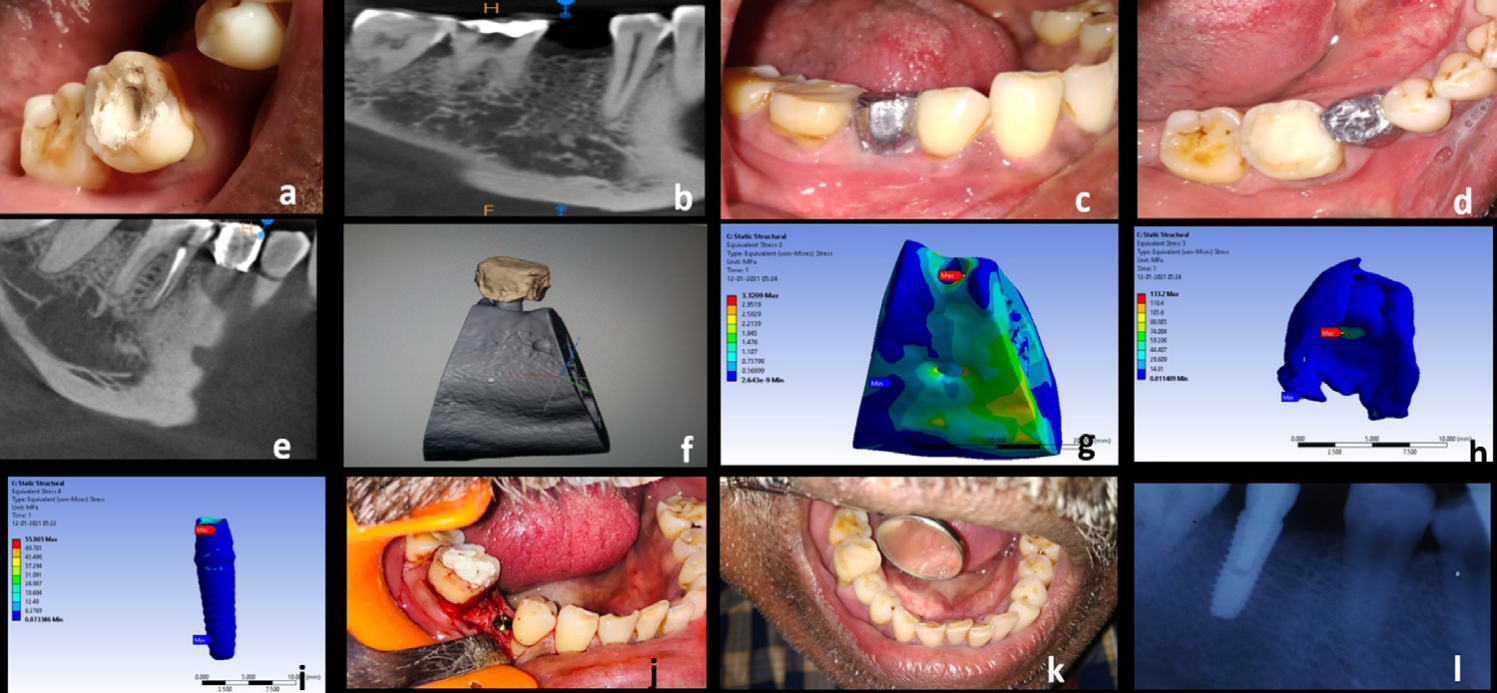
Clinical application process of implant supported single tooth rehabilitation in a respective patient, using lead foil crown delineation technique. a) Single missing tooth. b) CBCT of single tooth missing region. c) Removable prosthesis with countered led foil – buccal view. d) Removable prosthesis with countered led foil – occlusal view. e) BCT delineating the radiopaque lead foil crown. f) 3D model generated. g) FE analysd model of alveolar bone. h) FE analysed model of tooth crown. i) FE analysed model of dental implant. j) Implant placement. k) Final prosthesis on implant. l) Post operative radiograph.
